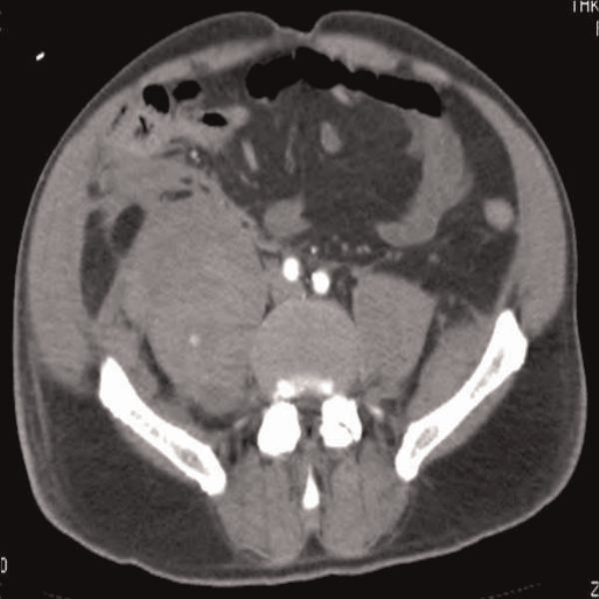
Abdominal angio-CT scan: bilateral iliopsoas haematomas.
